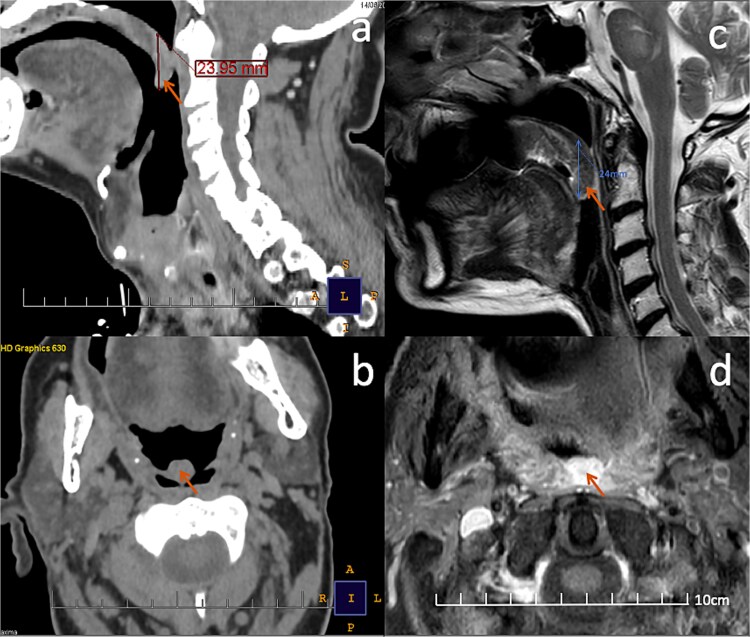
Comparison of pre-operative CT and post-operative MRI. (a, b) sagittal and axial contrast-enhanced CT images demonstrating an elongated uvula (orange arrow) measuring 23.95 mm vs 24 mm in length. (c, d) post-operative sagittal T2-weighted and axial post-contrast T1-weighted MRI acquired through the oropharynx. The uvula (orange arrow) shows homogeneous enhancement without focal mass effect, supporting its benign nature. The length of the uvula remains comparable on both modalities (a vs c); however, a slight increase in transverse diameter is appreciable on axial images (b vs d), likely related to reactive post-surgical inflammatory changes rather than recurrent tumor.
